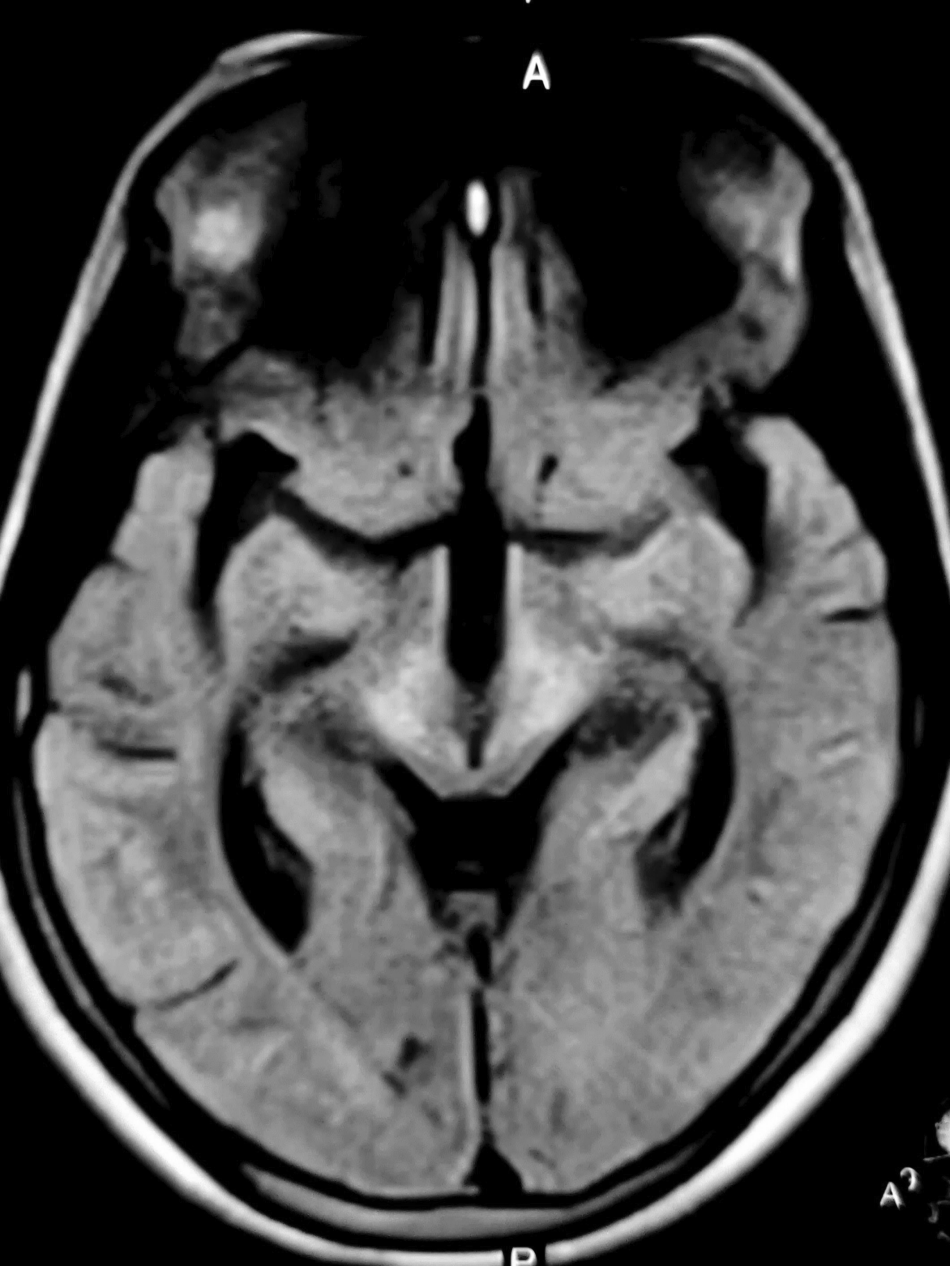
FLAIR hyperintensity in the midbrain.
